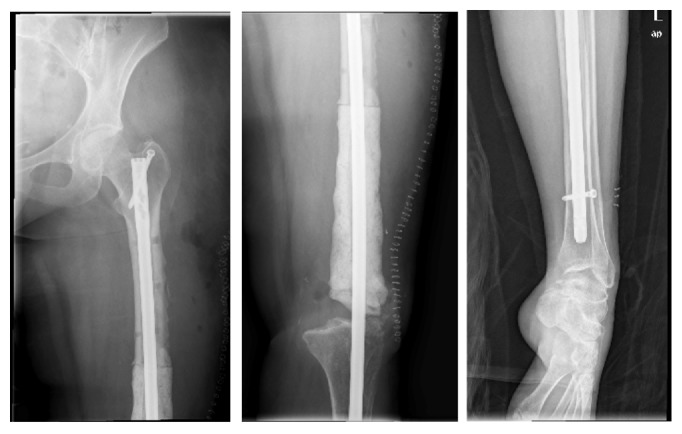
Postoperative radiographs with long IM nail and surrounding bone cement.
